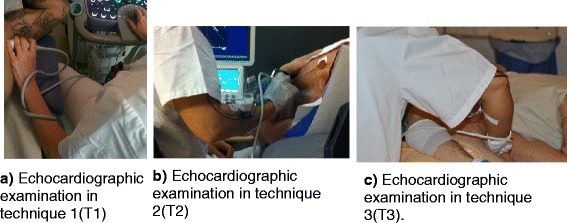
Pictures showing the three main echocardiographic examination techniques: Text to each picture: a T1, patient facing examiner, transducer in left hand, b T2, patient facing examiner, transducer in right hand, and c T3, patient facing away from examiner transducer in right hand
